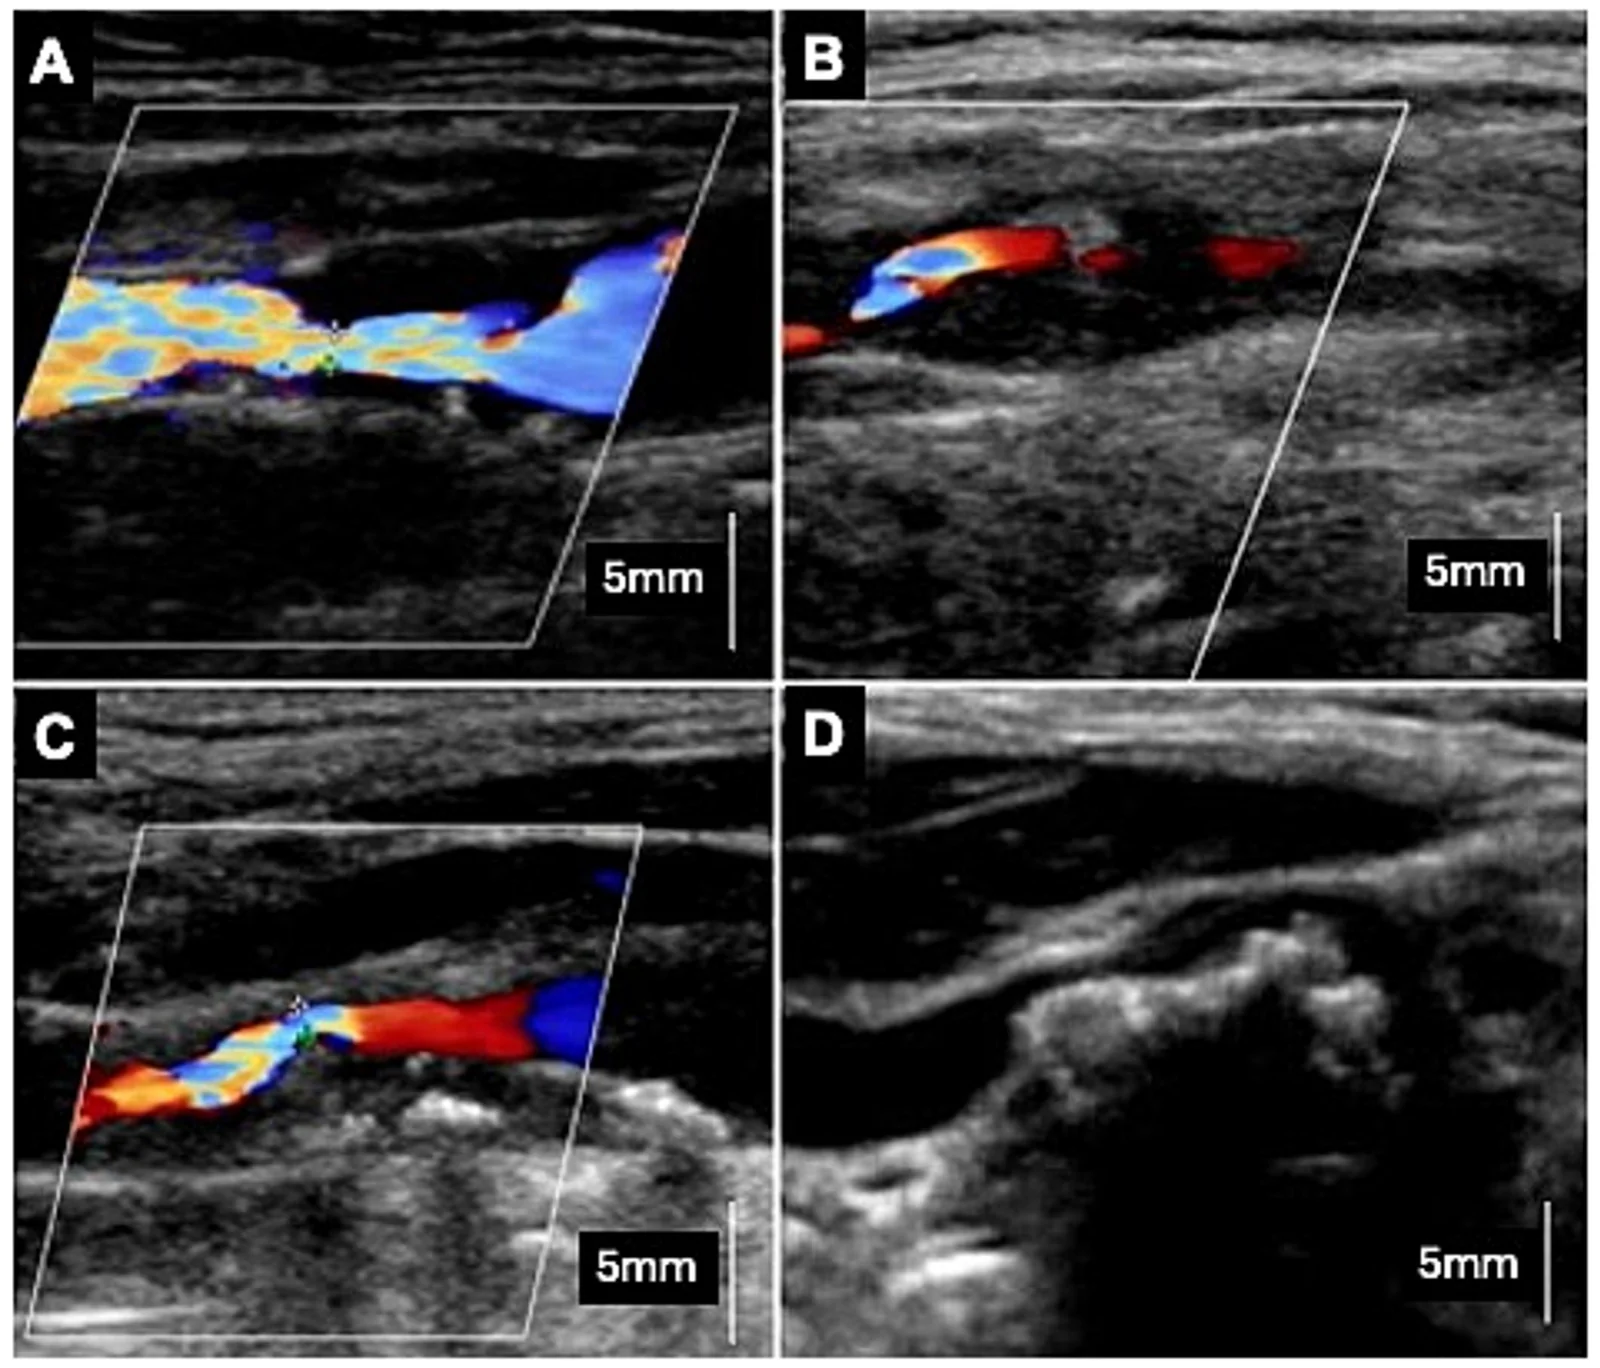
Carotid plaque echogenicity on ultrasound. Four patients with carotid stenosis comprised of plaques that are echolucent (A), more echolucent than echogenic (B), more echogenic than echolucent (C), and echogenic with acoustic shadowing (D) on ultrasound.
